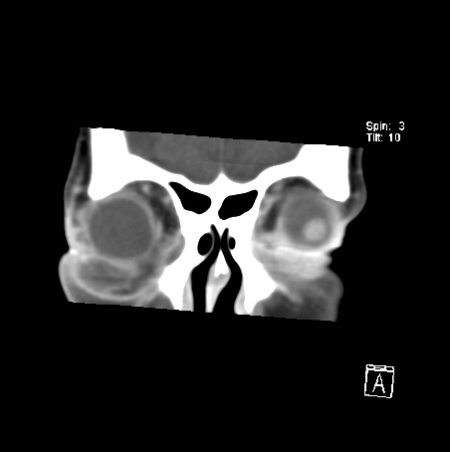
CT scan of the orbits and brain, coronal view. The swelling extended into the soft tissues of the infra-orbital region, but there was little pre-septal soft tissue swelling.
